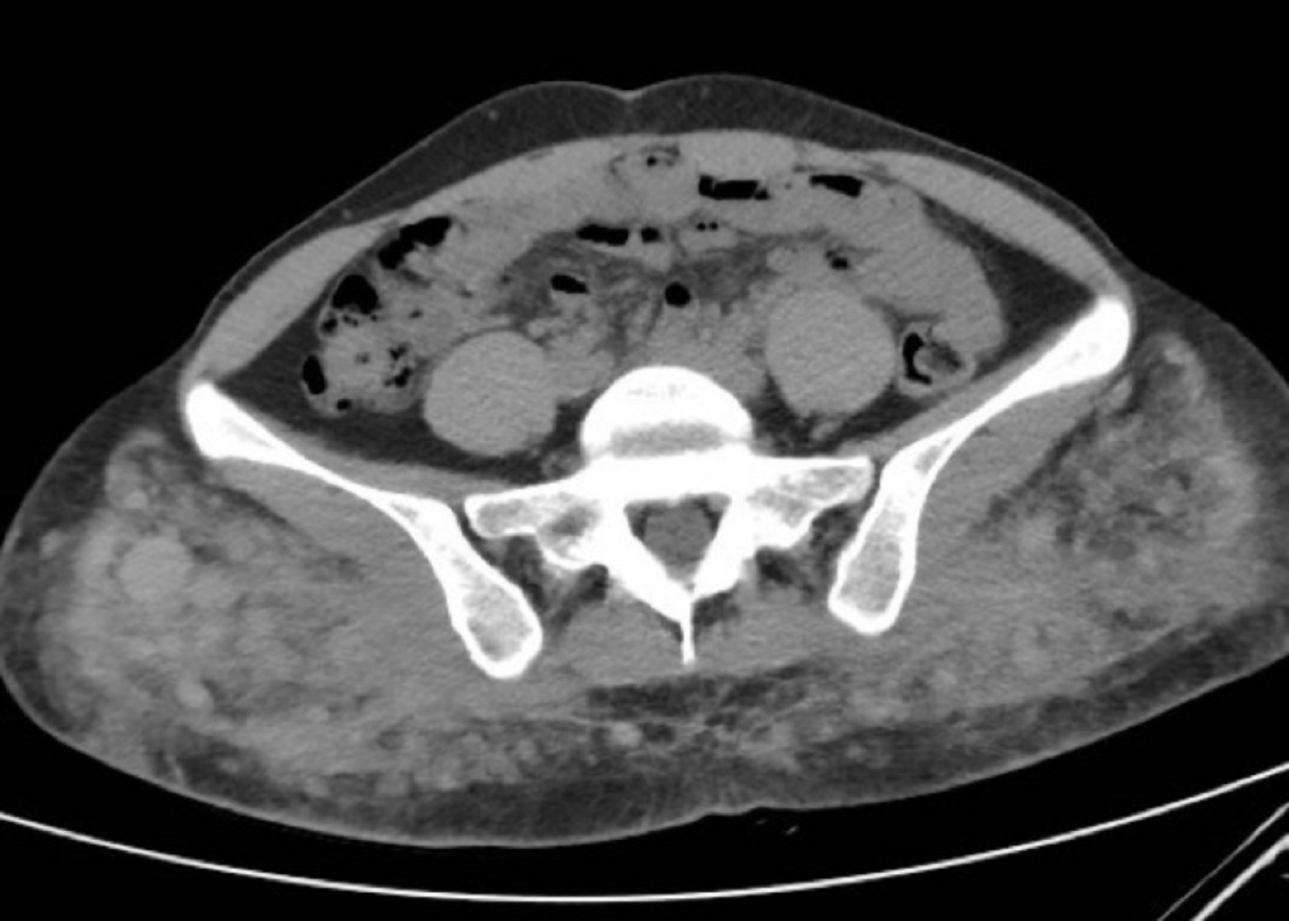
CT Abdomen/Pelvis Axial CT image of the pelvis demonstrates sites of prior subcutaneous gluteal free-silicone injection with associated reactive soft tissue changes, including granuloma formation.
